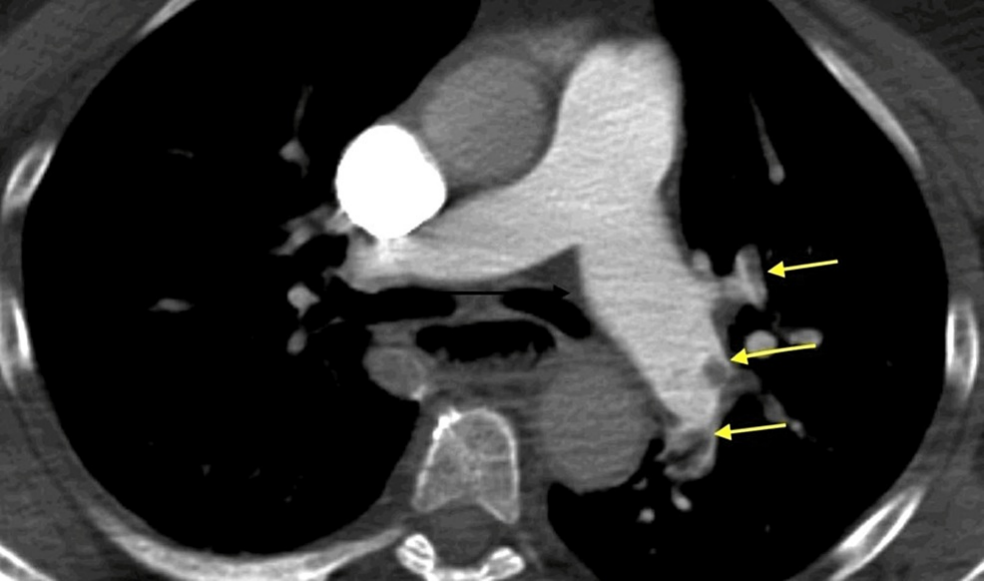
CT pulmonary angiogram with yellow arrows pointing at pulmonary emboli in the left pulmonary artery. CT, computed tomography
